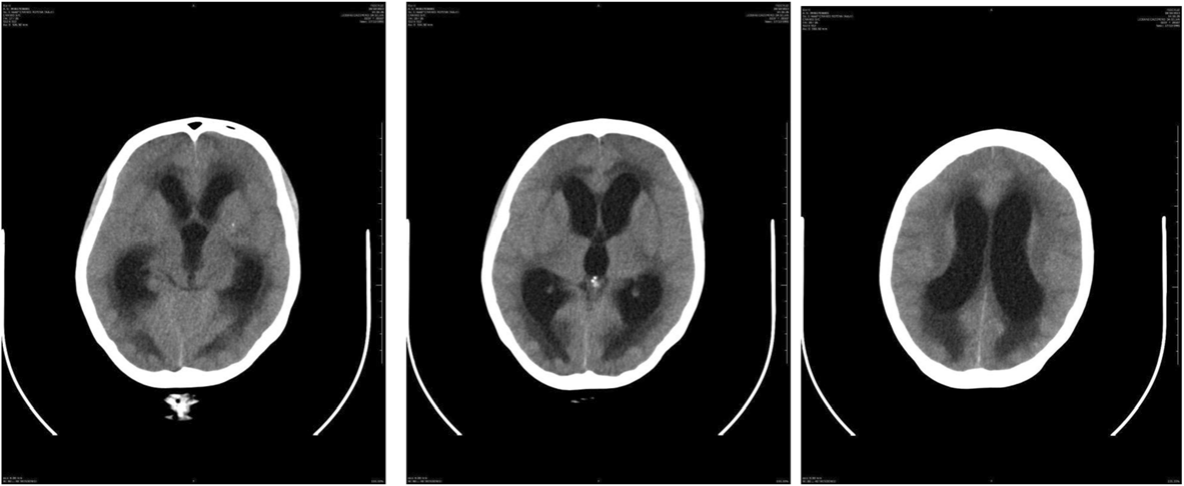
Brain CT Scan on October 2013 showing exudative hydrocephalus.
